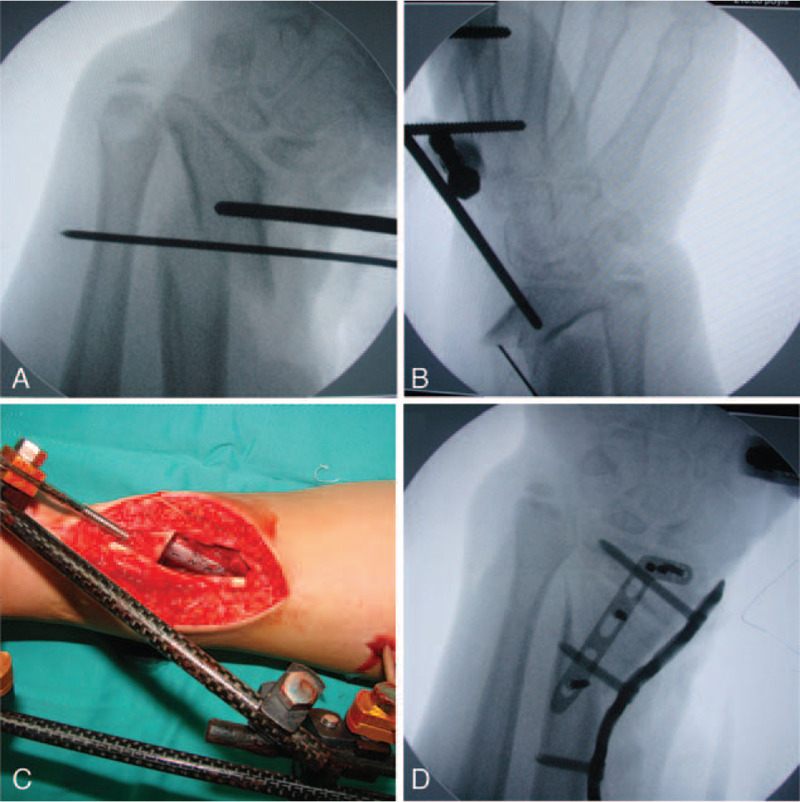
(A) A Schanz screw is employed to make the radiocarpal articular surface horizontal. (B, C) The external fixators are employed to fix the position of the Schanz screw. (D) Intraoperative radiographs confirmed the restoration of anatomy of the radiocarpal joint.
